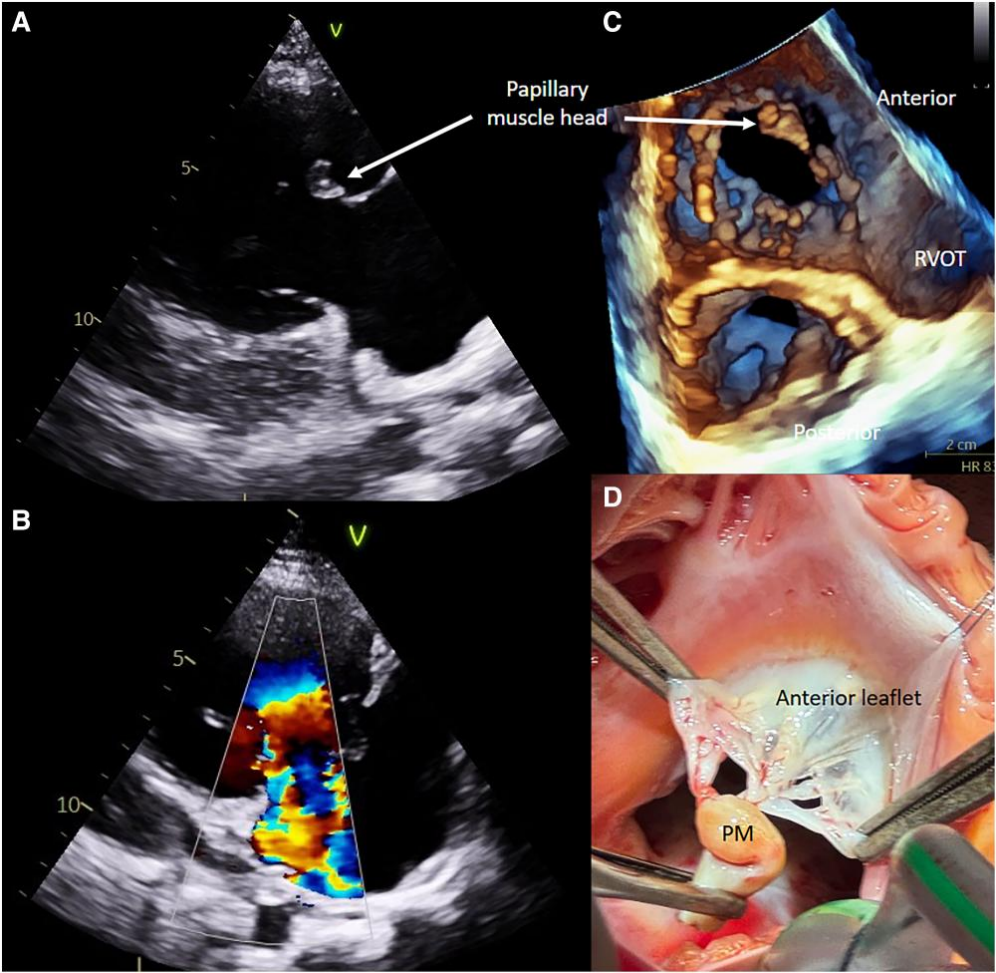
Echocardiographic and intraoperative views of traumatic tricuspid valve injury.
